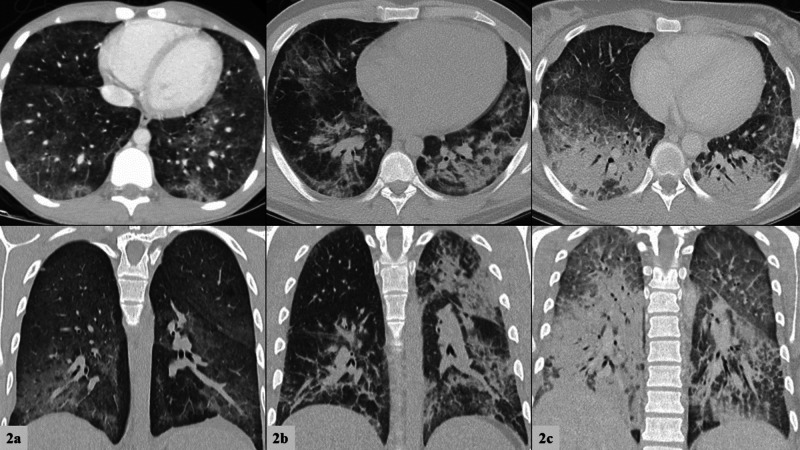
a-c: Initial computed tomography of the chest. Top row frames depict transverse views and bottom row frames depict coronal views. From left to right: Patient 1, Patient 2, Patient 3. 2a “Ill-defined fluffy confluent centrilobular nodules seen bilaterally, more confluent and dense the lung bases with subpleural sparing.” 2b “Bilateral patchy ground glass and consolidative opacities.” 2c “Dense bilateral lower lobe consolidations. Bilateral upper lobe and right middle lobe ground glass consolidations with interstitial thickening.”
